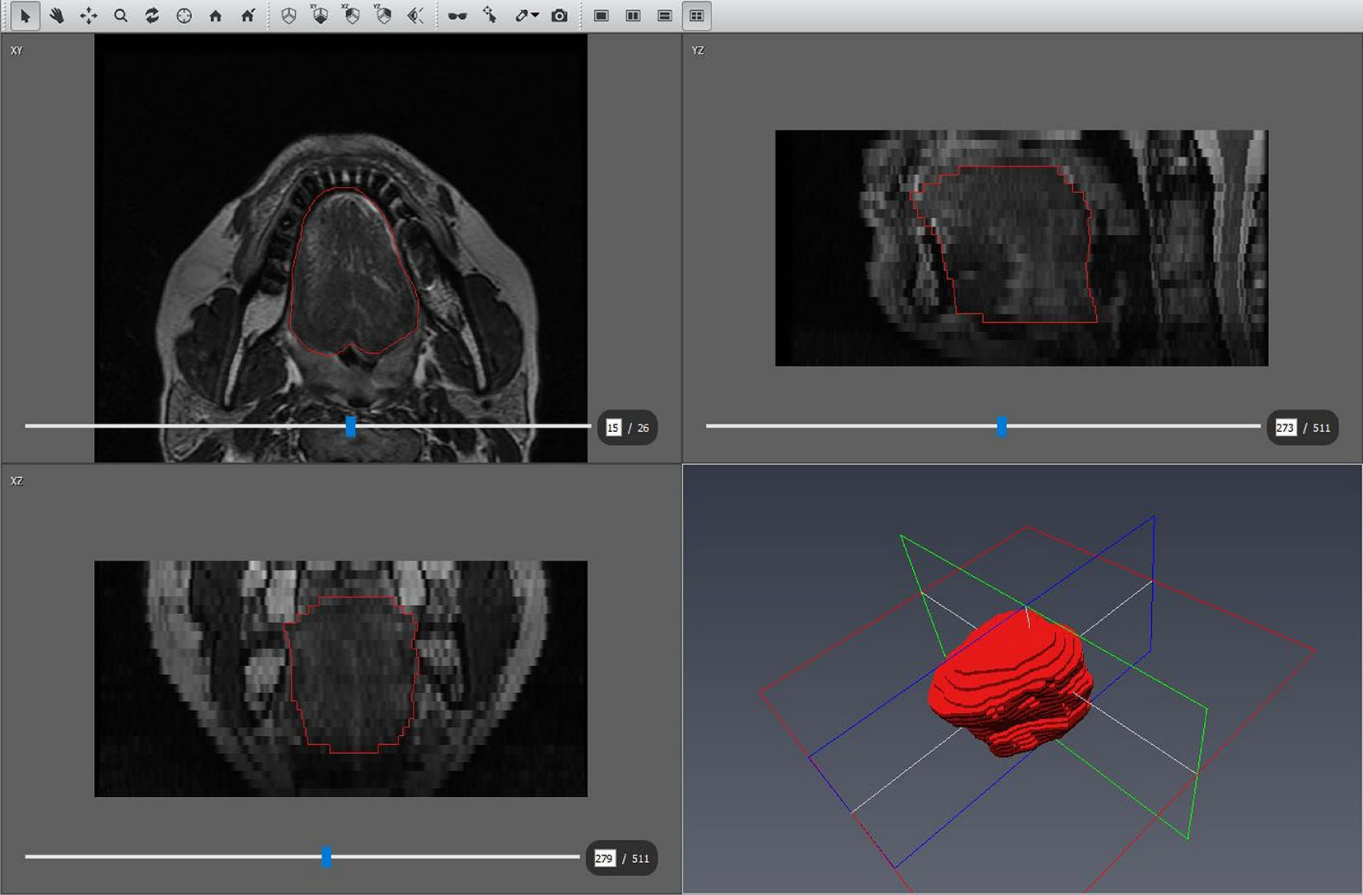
Tongue fat tissue volumetric analysis. Example screenshot of Amira image analysis software to segment the tongue and to measure total tongue volume (mL) on the in-phase T2-weighted images with multiplanar reformats. Mean signal in the tongue volume on the fat-only images (not shown) was divided by mean signal in parapharyngeal adipose tissue to yield an estimate of tongue fat fraction (%), which was then multiplied by total tongue volume to yield an estimate of tongue fat volume (mL) for each subject. Values are reported in Tables 1 and 2.
